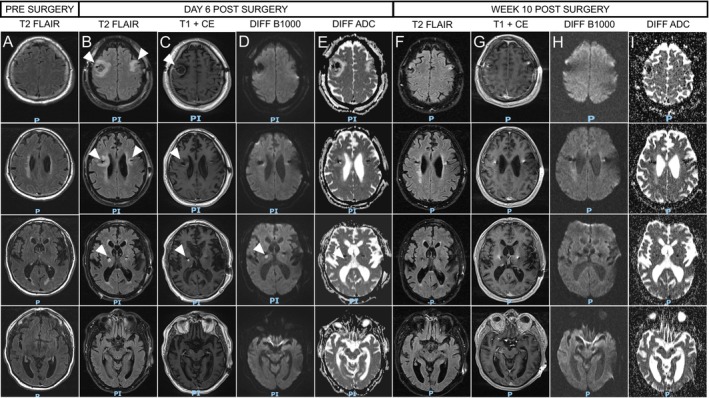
Longitudinal evaluation of brain MRI. (A) MRI pre surgery showing several microvascular white matter lesions. (B) Extensive edema as reflected by hyperintensities on T2‐weighted fluid‐attenuated inversion recovery (FLAIR) MRI on day 6 post‐surgery along the electrodes' trajectories, pronounced on the right side (marked with arrows). (C) Bilateral cortical annular contrast enhancement (CE) on both sides, marked on the right side (marked with arrows). (D) Diffusion weighted imaging (DWI) revealed no signal restriction in the area of annular CE (limited accessibility due to metal artifacts) but suggested right thalamic infarction (marked with arrow) with corresponding signal on the ADC‐map (E). (F) Follow‐up MRI at week 10 postsurgery showed near complete remission of edema on FLAIR imaging with complete remission of CE on T1‐weighted MRI (G). There was no sign of (chronic) ischemic infarction in the right thalamus (H, I).
